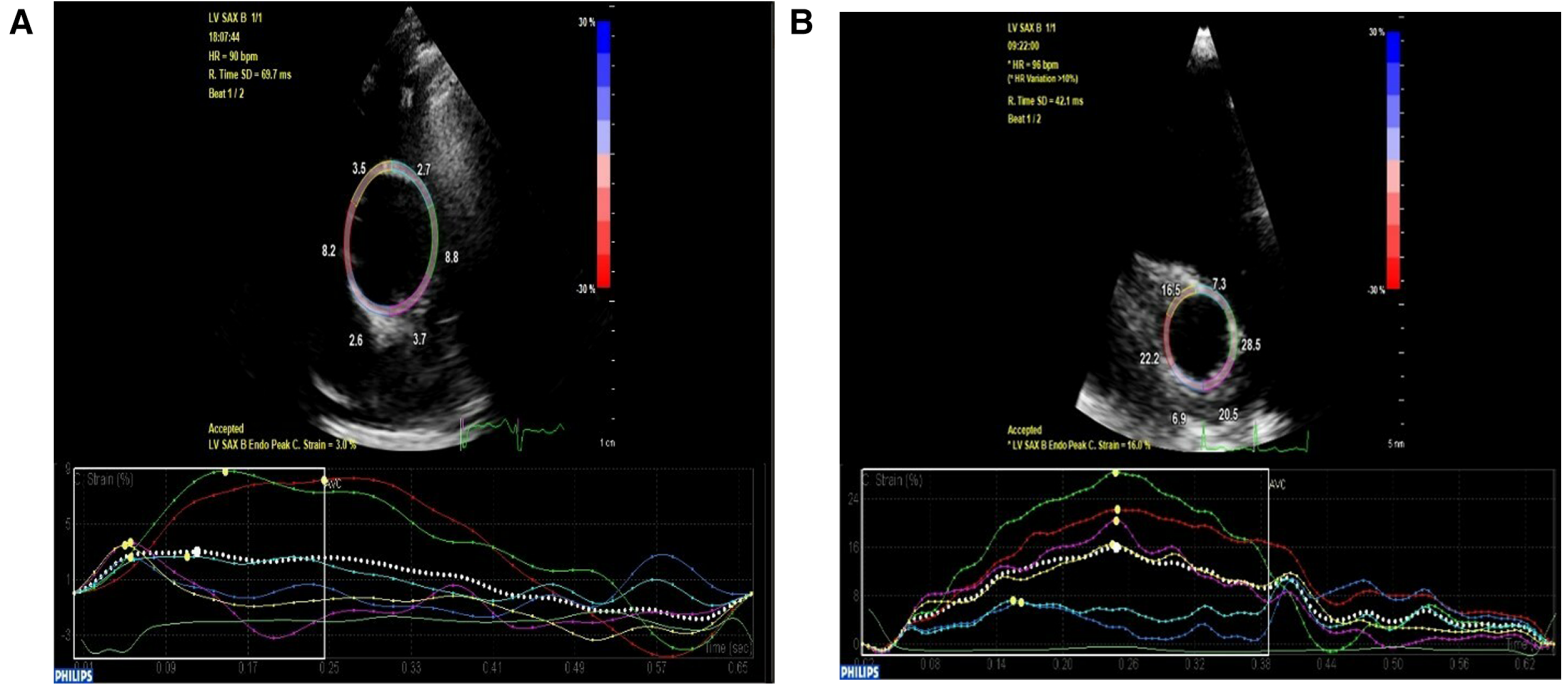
High parasternal short axis view of the ascending aorta depicting decreased circumferential strain at 3% in a patient with ascending aortic aneurysm (A) compared to age and gender-matched normal control with preserved circumferential strain at 16% (B).
